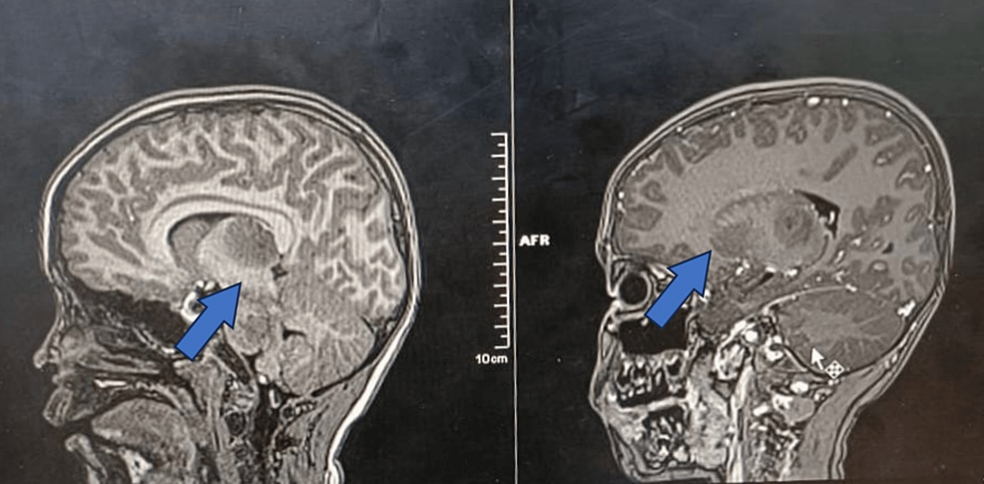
Saggital plane MRI shows swelling and symmetrical abnormal signal intensities involving bilateral thalami (arrows).
